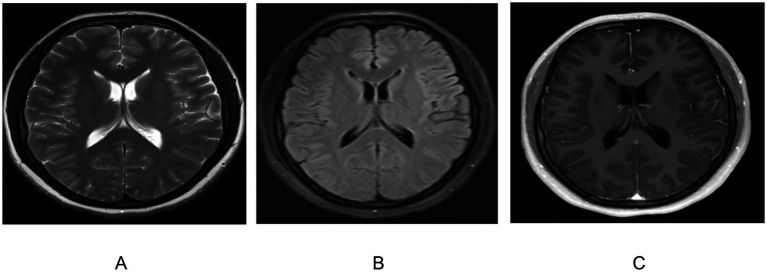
Brain magnetic resonance imaging (MRI) was performed after admission: (A) T2WI; (B) T2FLAIR; (C) Enhanced MRI.
